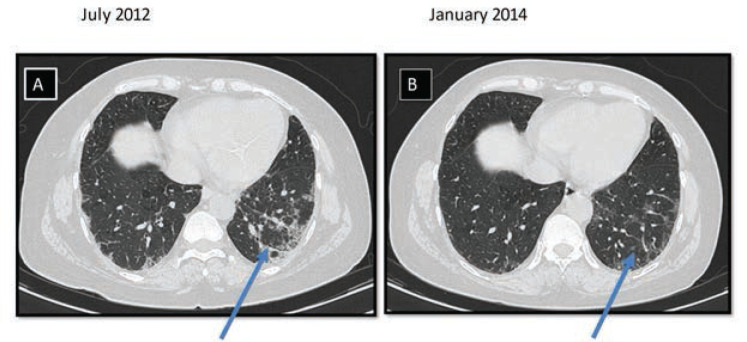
High-resolution CT thorax of a patient at baseline (A) and after 2 years of treatment (B). (A)Shows microcystic honeycombing, bronchiectasis, and traction bronchiectasis at the basal zones of the lung with involvement of subpleural region (B) marked resolution in the lung parenchymal changes after treatment
